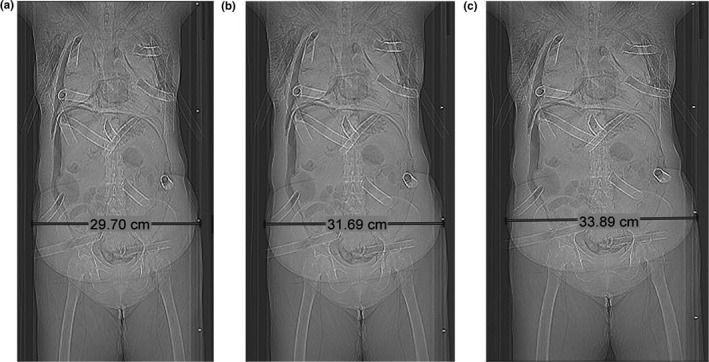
Localizer radiograph images of Subject 3 (a) shifted 4 cm posteriorly, (b) centered, and (c) shifted 4 cm anteriorly.
